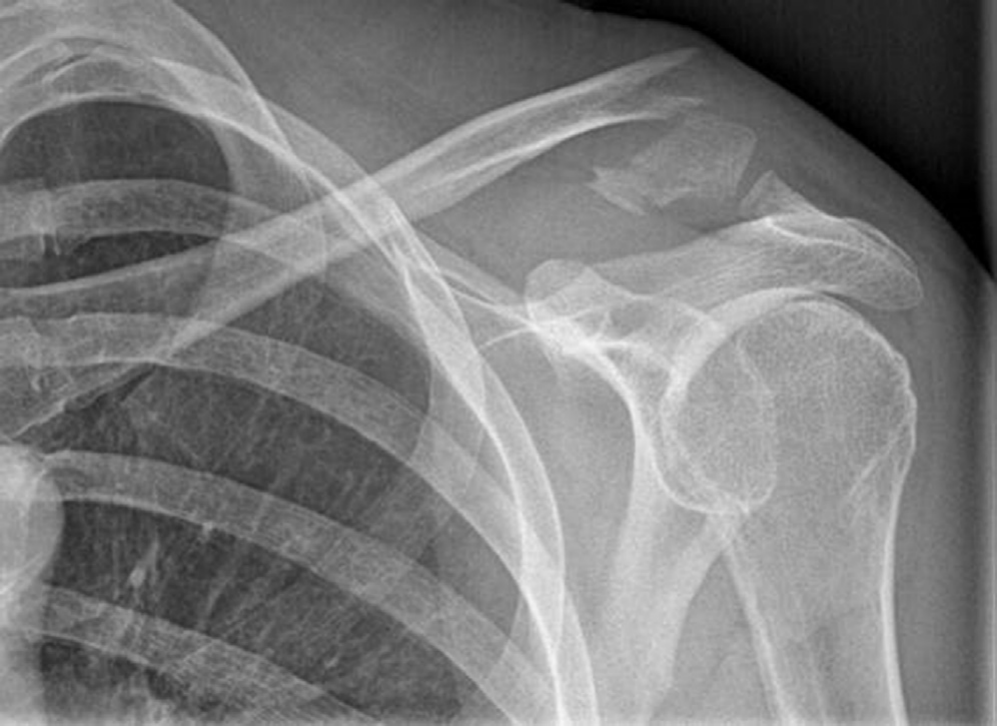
Radiograph of patient with Neer type 2 fracture.
